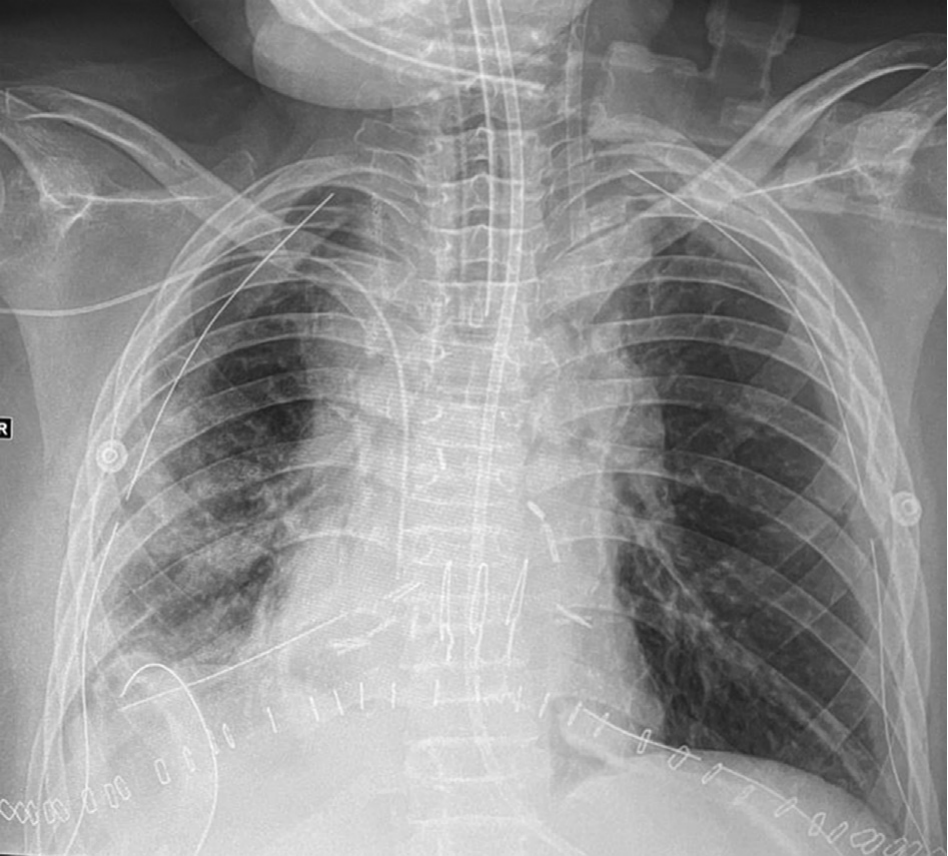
Postoperative radiograph. Postoperative radiograph taken on postoperative day 2 shows good-size matching and aeration of both lung grafts.
